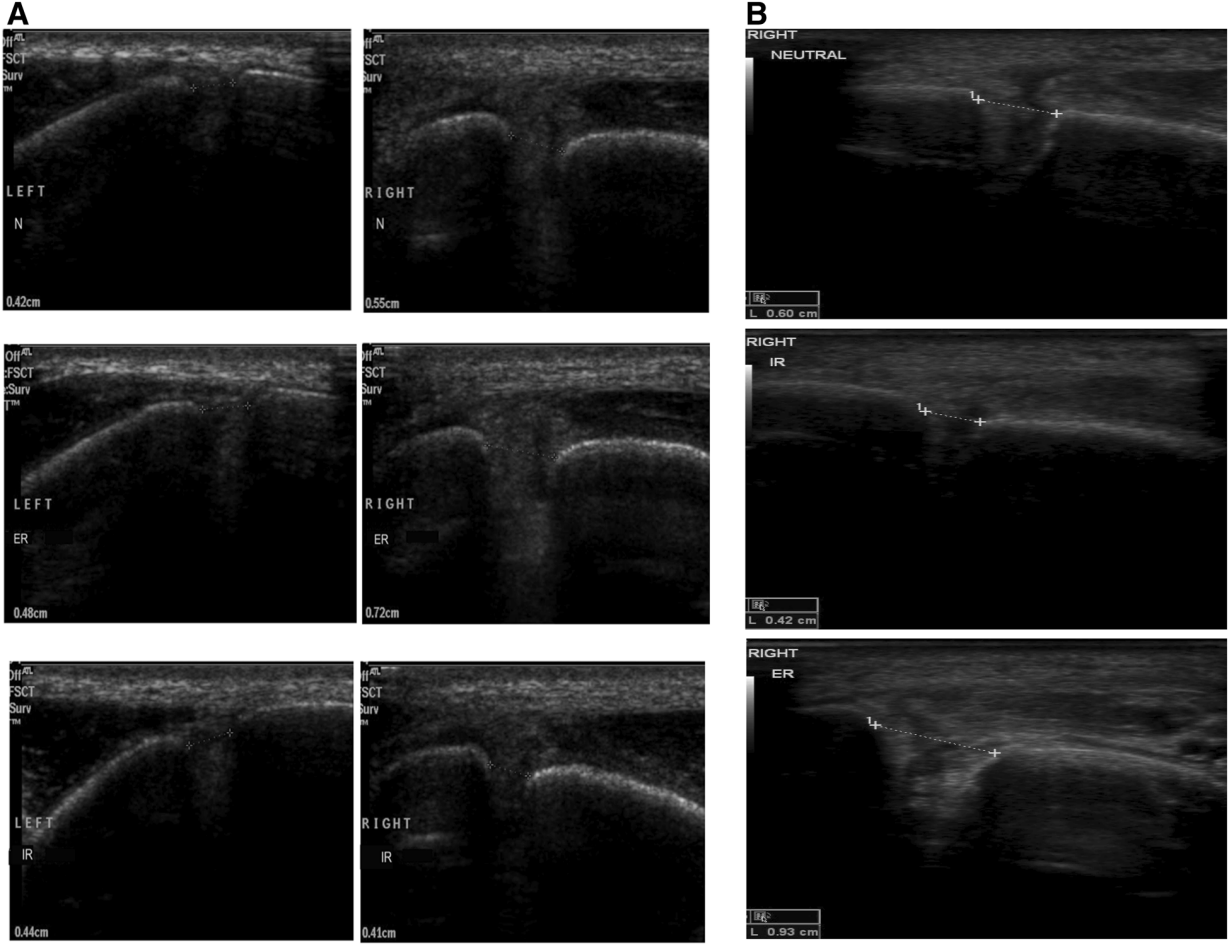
Dynamic Ultrasound views of the Left clear space with healthy AITFL and the Right clear space with torn AITFL, of the same patient. A: The healthy ligament is seen connecting the tibia to the fibula and with only a minimal measured clear space opening under stress (ER=External Rotation, IR=Internal rotation, N=Neutral). The right torn side shows a marked clear space opening under stress (ER) as there is no continuous ligament to stop separation of the tibia and fibula. Application of Internal Rotation stress tightens the joint, bringing the tibia and fibula closer to each other, in the absence of the AITFL. B: Dynamic Ultrasound views of a Torn AITFL which presents with widened clear space. (ER=External Rotation, IR=Internal rotation, N=Neutral). The injured syndesmosis shows a marked clear space opening under stress (ER) as there is no continuous ligament to stop separation of the tibia and fibula. Application of Internal Rotation stress tightens the joint, bringing the tibia and fibula closer to each other, in the absence of the AITFL.
